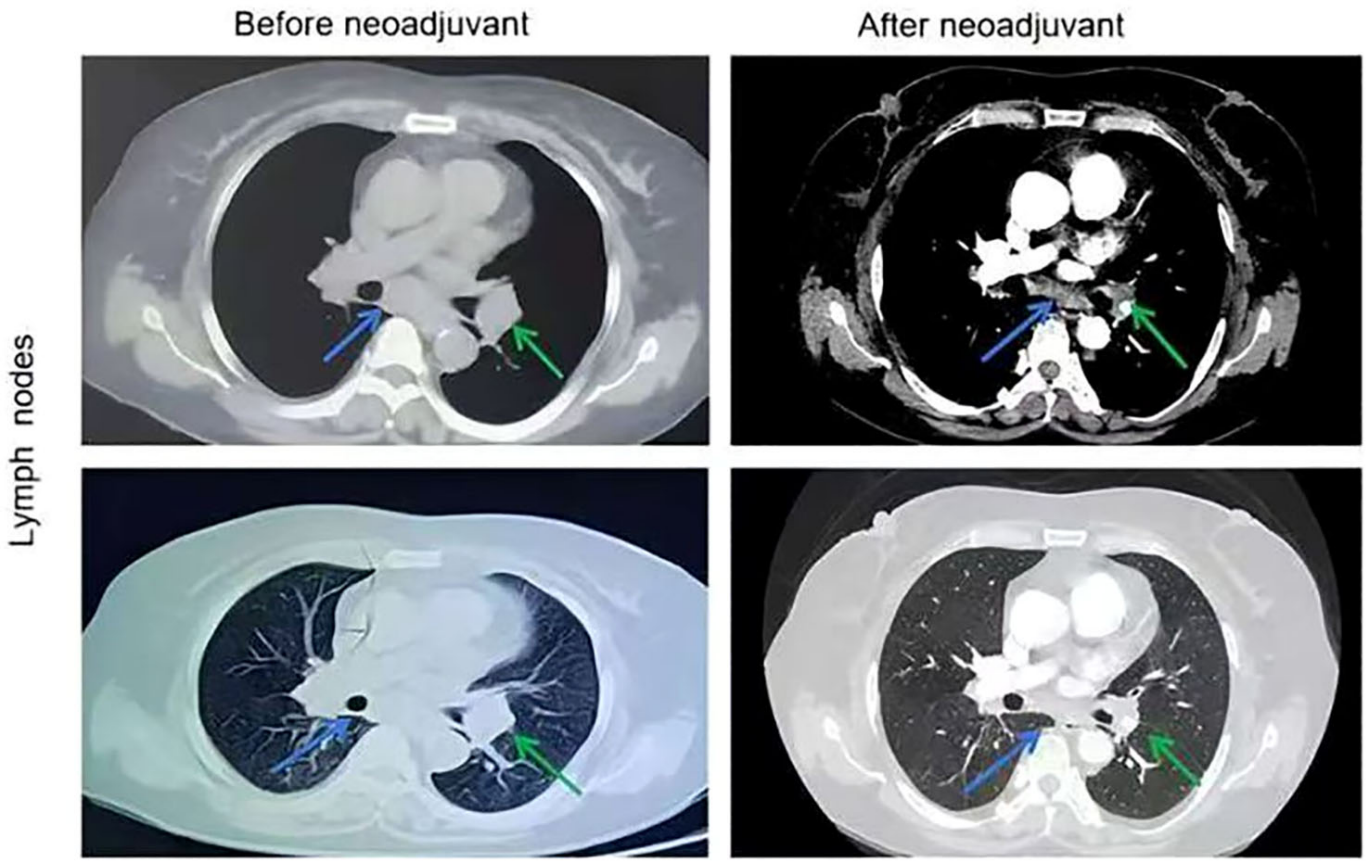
Chest CT images of lymph nodes before and after the neoadjuvant therapy. The stations 7 lymph nodes pointed by blue arrows and the stations 10 lymph nodes pointed by green arrows.
